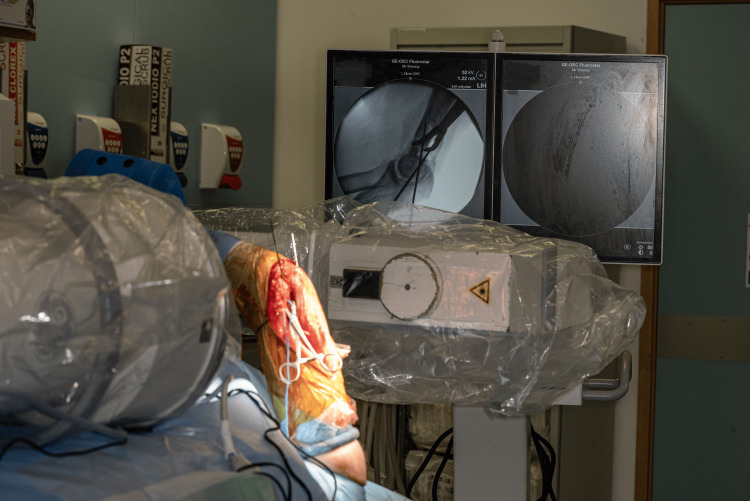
Image demonstrating a true lateral radiograph of the elbow. The proposed technique allows for true lateral views of the elbow without the need for the surgeon or an assistant to maintain the positioning of the arm.
